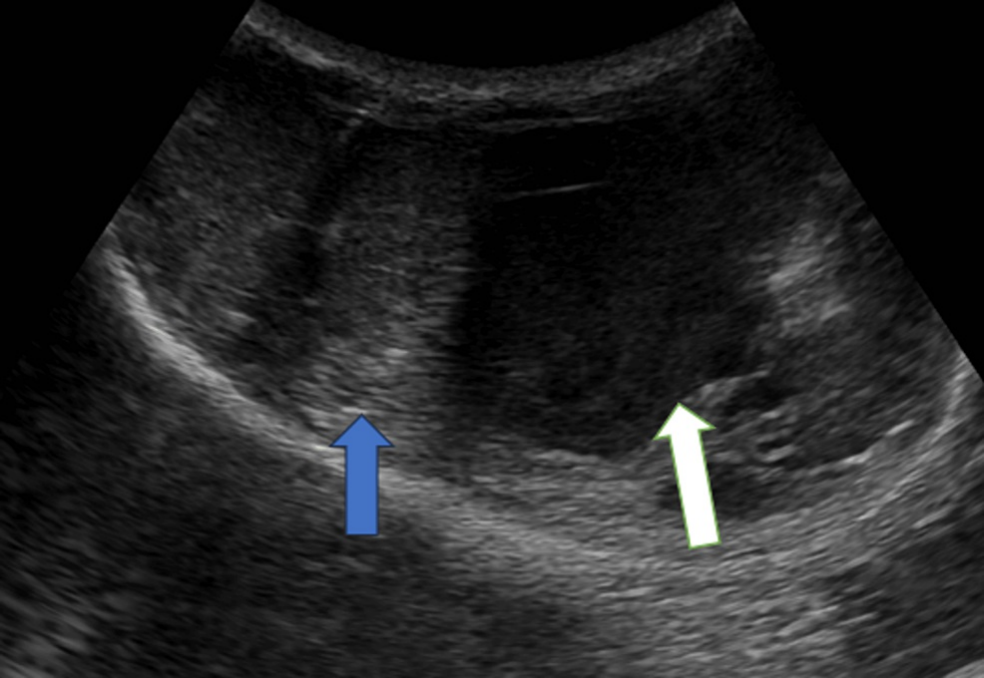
Ultrasound image of the scrotal sac using curvi-linear probe showing hypoechoic collection in the left scrotal sac (white arrow) with a displacement of the left testes away from the midline toward the right (blue arrow).
